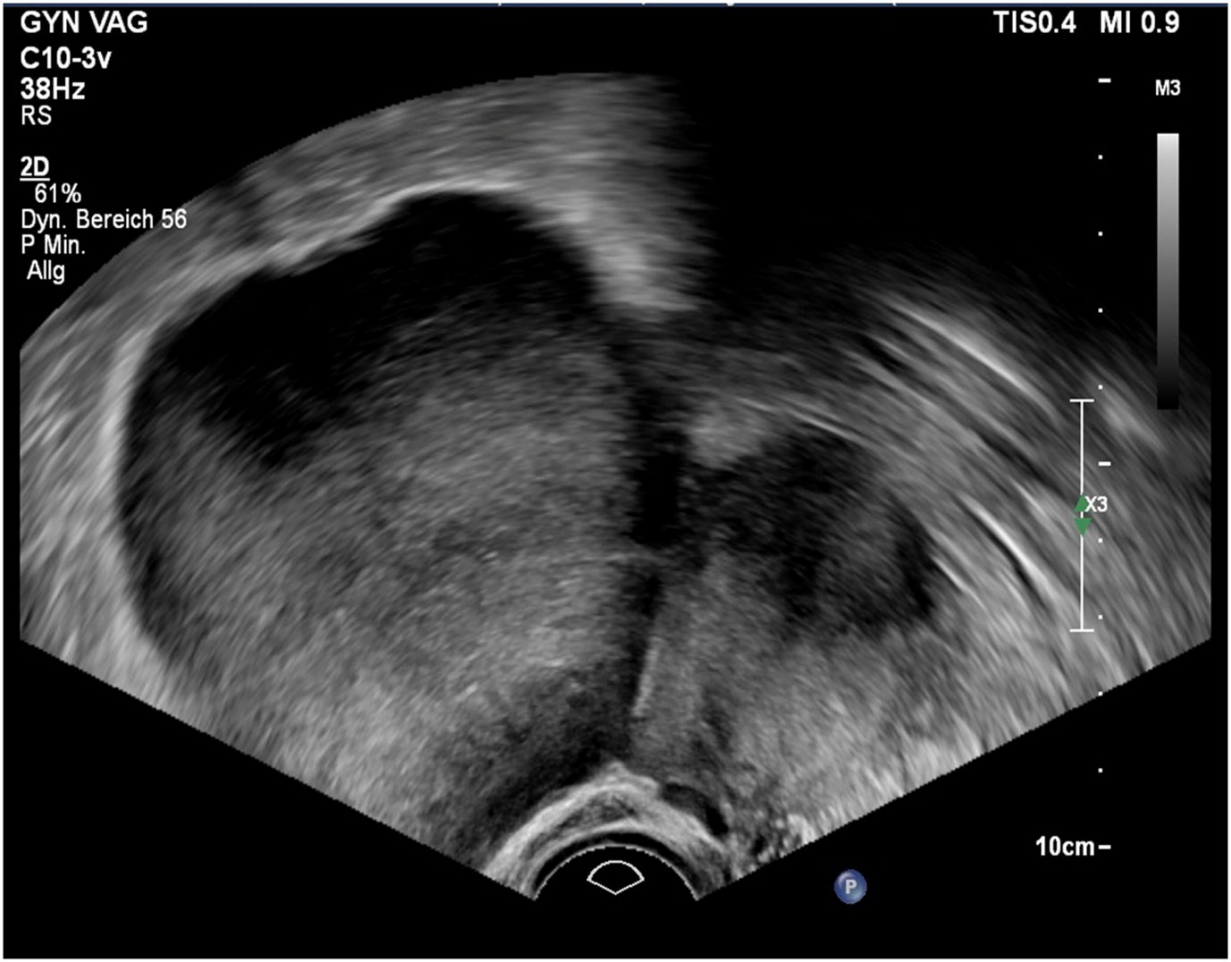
Transvaginal ultrasound image of dysgerminoma of left ovary (diameter 9,8 cm).
